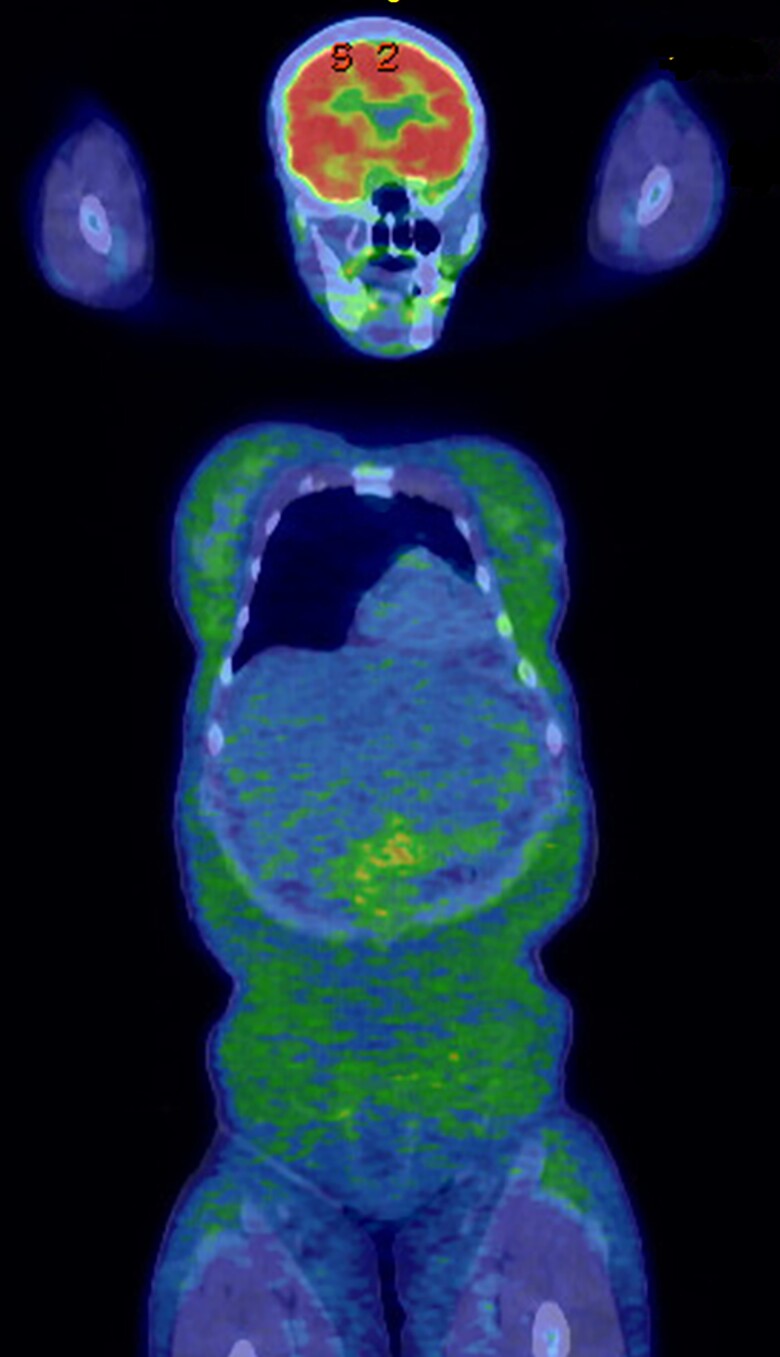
Whole body FDG-PET scan showing diffuse mild subcutaneous FDG activity suggestive of panniculitis. Abbreviations: FDG, fluorodeoxyglucose; PET, positron emission tomography.
